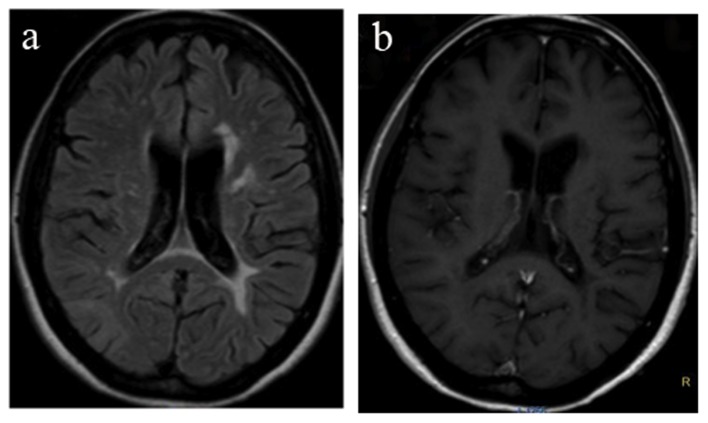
MRI 2 months after demonstrating a resolution of the lesion of the right parietal lobe (a) and absence of gadolinium enhancement (b).
